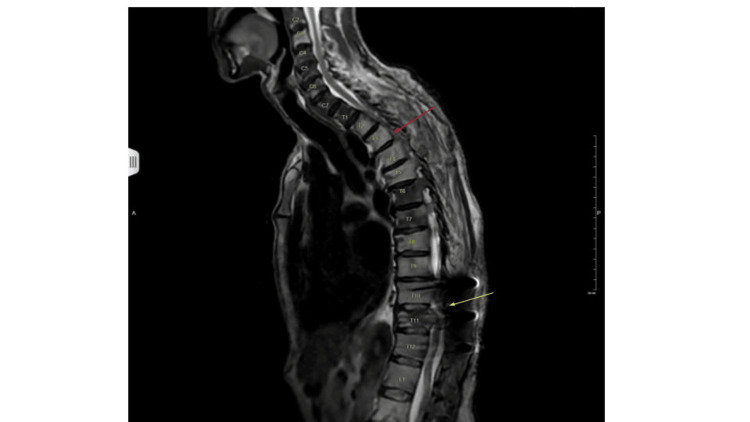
Postoperative MRI of the spine after T2-T4 laminectomy (red arrow) and T10-T11 laminectomy with tumor debulking and T10-T12 posterior instrumented fusion (yellow arrow)
